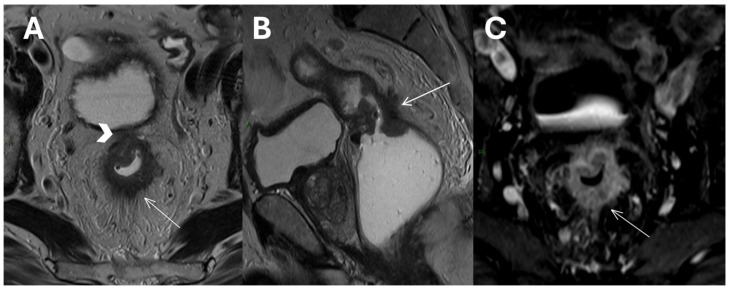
T2-weighted Turbo Spin Echo images in axial (A) and sagittal (B) planes of a mid-upper rectal tumor showing invasion of the mesorectal fascia (arrowhead), perirectal tumor invasion, and desmoplastic stranding (white arrows). An axial T1 Volumetric Interpolated Breath-hold Examination image after contrast agent administration (C).
